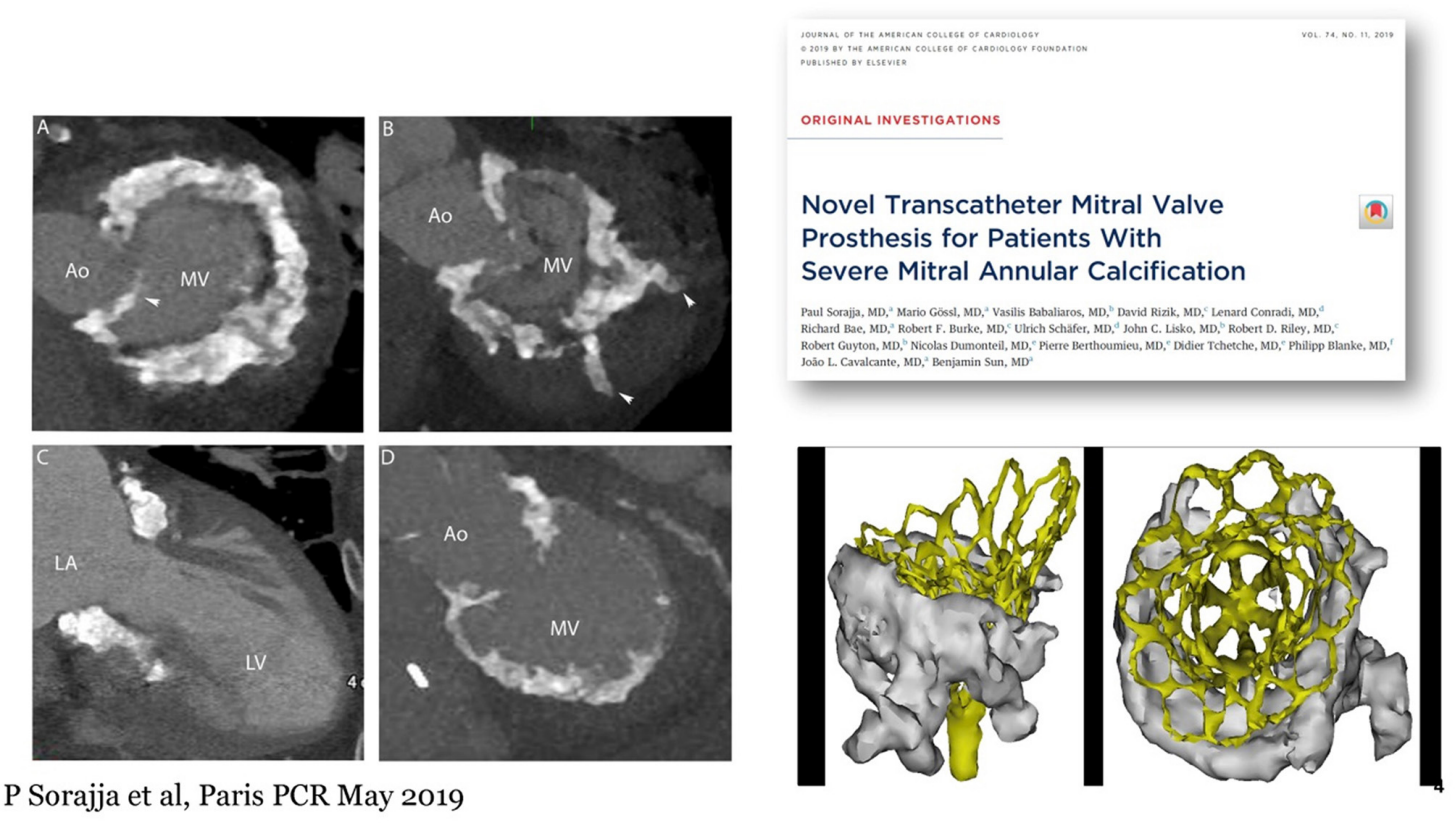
Experience of Tendyne in MAC. First Presented by Sorajja et al. (20). Left: Representative Patterns of Severe MAC in Patients With Symptomatic MR Images from gated, contrast-enhanced cardiac computed tomography are shown. (A,B,D) are from unique patients, whereas (A,C) are from the same patient. Some patients had large spicules arising from the anterior horn of the mitral annulus (A, arrowhead) or invading the myocardium (B, arrowheads). Ao, aorta; LA, left atrium; LV, left ventricle; MAC, mitral annular calcification; MR, mitral regurgitation; MV, mitral valve. Right: Tendyne implanted in MAC.
